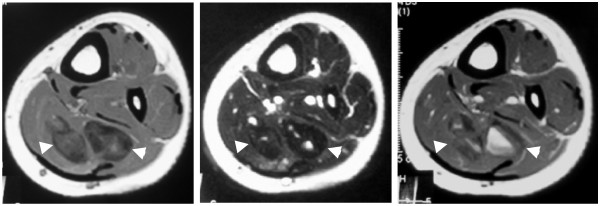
Magnetic resonance images of the lesion. T1-weighted images showed a mixture of areas of isointense and low signal intensity (left panel). T2-weighted images showed areas of low signal intensity (middle panel). On gadolinium-enhanced T1-weighted images, the lateral mass showed significant enhancement (right panel).
